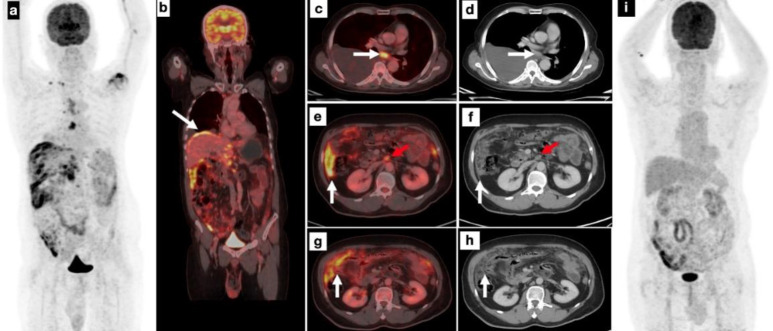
Maximum intensity projection (MIP) image of the baseline ^18^F-FDG PET/CT (a) demonstrates multiple hypermetabolic foci in the right lower cervical, mediastinal, and abdominal regions. Coronal fused PET/CT images (b) reveal increased FDG uptake along the peritoneal lining, particularly over the liver capsule. Axial fused PET/CT and corresponding CT images show right-sided pleural effusion and FDG-avid subcarinal lymph node (c, d), retroperitoneal lymphadenopathy (e, f, red arrow), and intense FDG uptake along the omentum and peritoneum (e, g, white arrow). Physiological FDG uptake is noted in the ascending colon, and focal uptake in the left shoulder region indicates bursitis (a). Follow-up ^18^F-FDG PET/CT after empirical anti-tubercular therapy shows complete metabolic resolution of previously observed lesions (i), confirming a favorable treatment response
